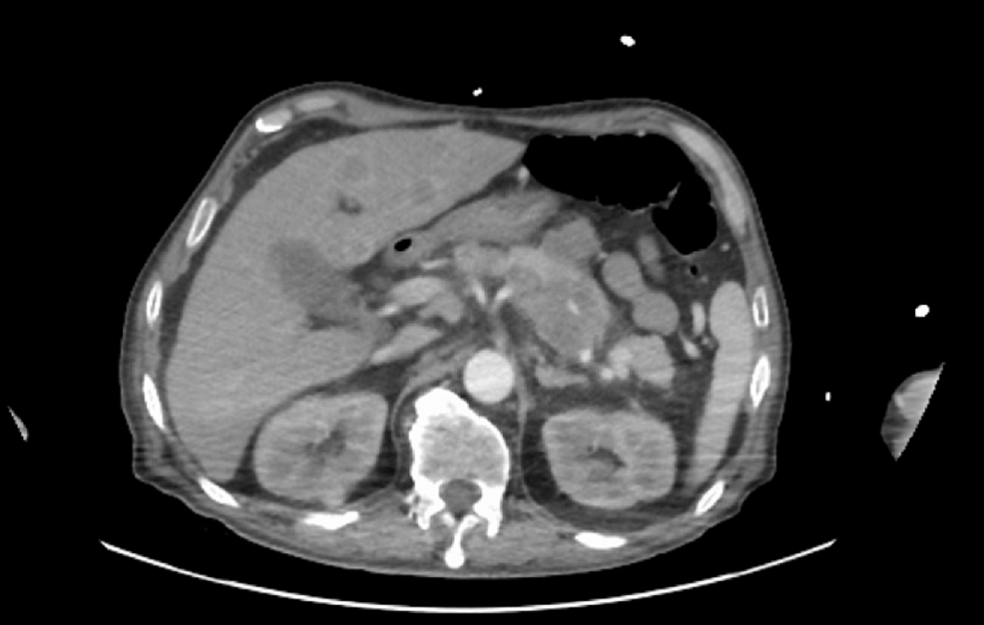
CT of the abdomen with contrast. Multiple hypodense ill-defined lesions throughout the liver compatible with metastatic disease. Extensive necrotic adenopathy involving the upper abdomen adjacent to the stomach and caudate lobe that measures at least 6x8.3 cm that extends into the body of the pancreas.
